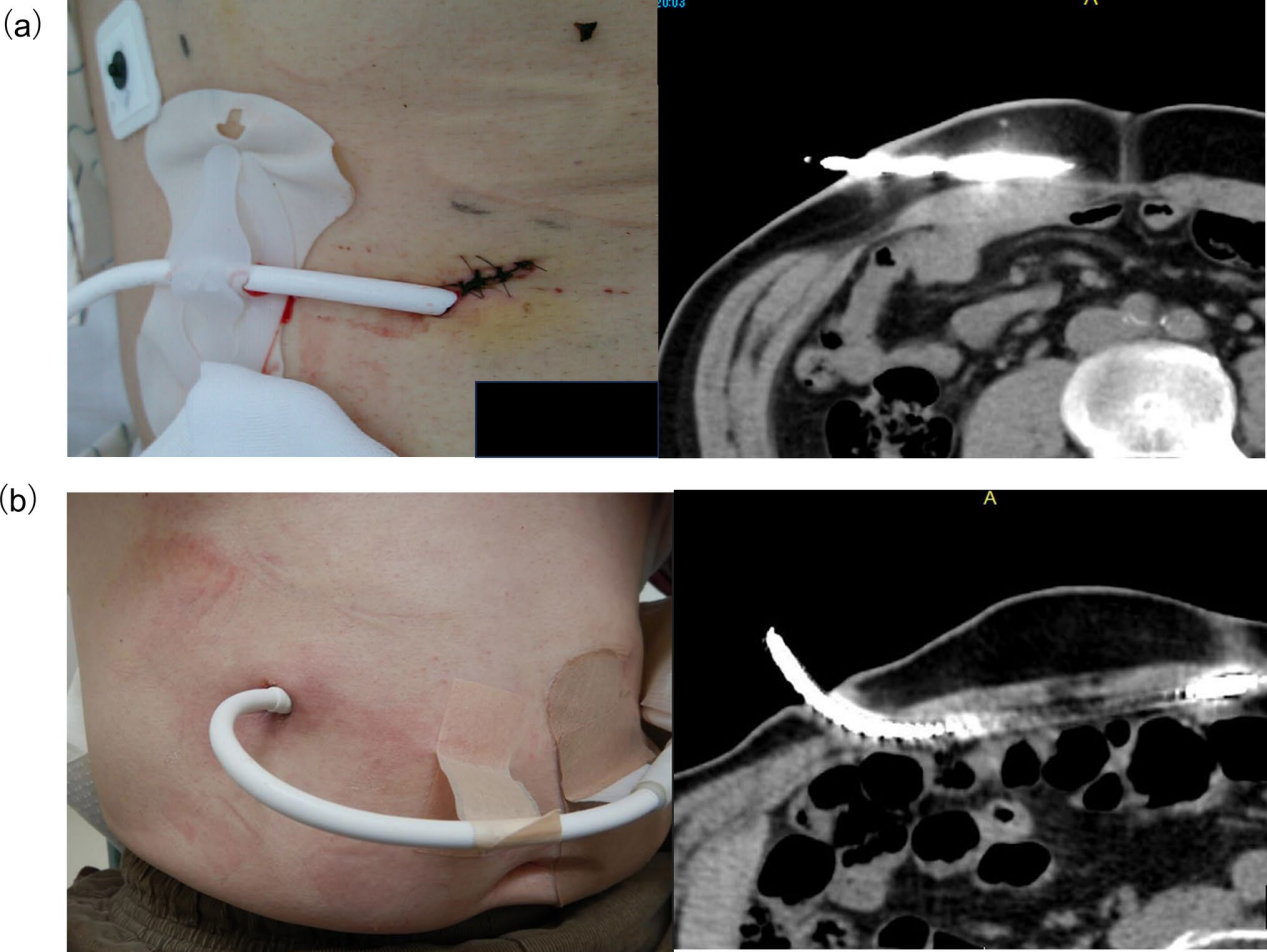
Comparison of conventional driveline management and modified fixation method. a Picture and computed tomography images of skin penetration of the LVAD driveline in a patient with conventional driveline management. b Picture and computed tomography images of skin penetration of the LVAD driveline in a patient with the modified fixation method
